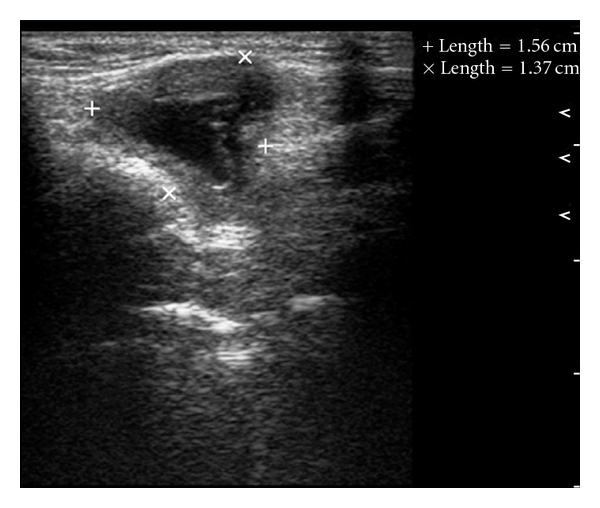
Longitudinal grey-scale ultrasound in the left submandibular region shows an anechoic TDC with thick wall.
